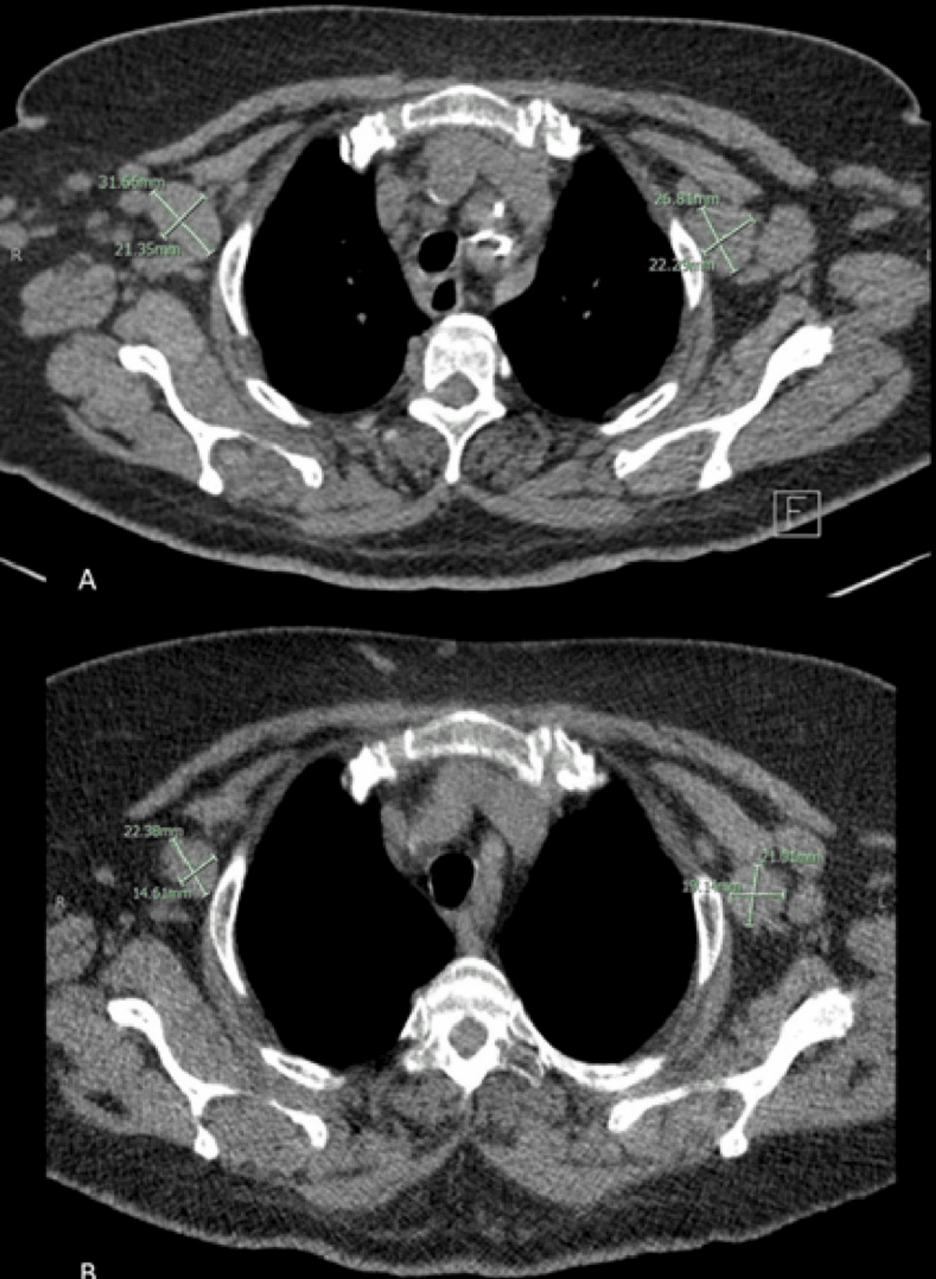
High-resolution CT scan of the chest showing multiple enlarged lymph nodes in the neck (a) before tocilizumab and (b) decrease in size after treatment with tocilizumab
